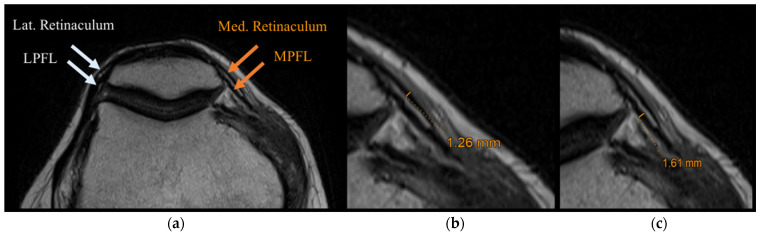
Radiological study: (a) MRI with MPFL, medial retinaculum, LPFL, lateral retinaculum indicated; (b) measurement of the medial retinaculum; (c) measurement of the medial patellofemoral ligament.
